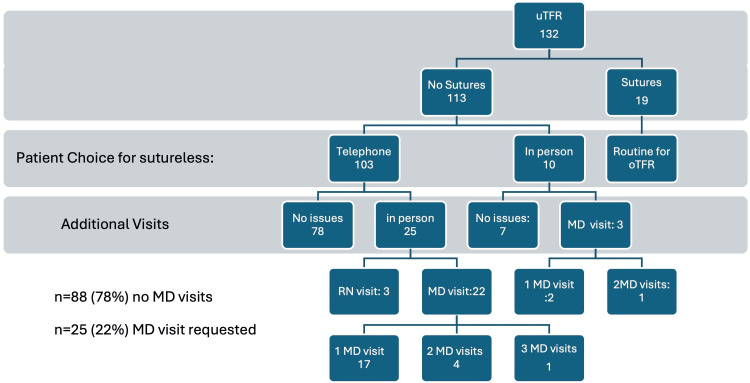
Postoperative course for uTFR only patients. uTFR: ultrasound trigger finger release; RN: registered nurse; MD: medical doctor
